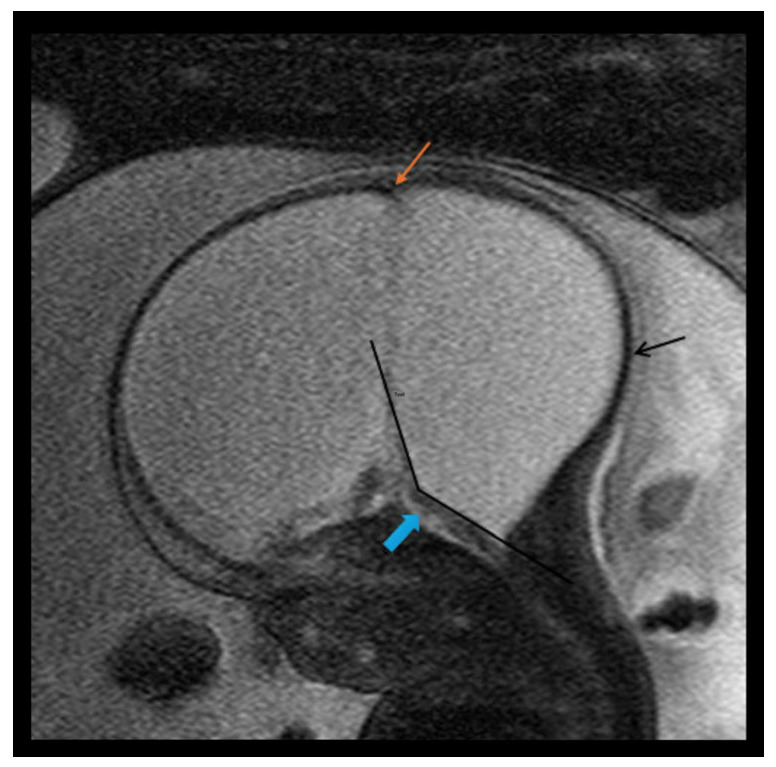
Fetal MRI image of a fetus at 31 gestational weeks with a classical Dandy–Walker cyst and massive hydrocephalus with a possible form of encephaloclastic schizencephaly. The T2-weighted sequence in the sagittal plane depicts a large posterior fossa cyst representing massive enlargement of the 4th ventricle as part of a large classical Dandy–Walker cyst. Pictured is an enlarged intracranial cavity occupied almost completely by CSF, a large posterior fossa cyst with ballooning of the occiput (black arrow), and a thin brainstem (blue arrow) with anterior displacement. There was no evidence of a cerebellum and there was elevation of torcular implantation (orange arrow) and an increased tegmento–vermian angle (black lines). There were enlarged lateral ventricles secondary to the massive hydrocephalus and/or a form of encephaloclastic schizencephaly.
